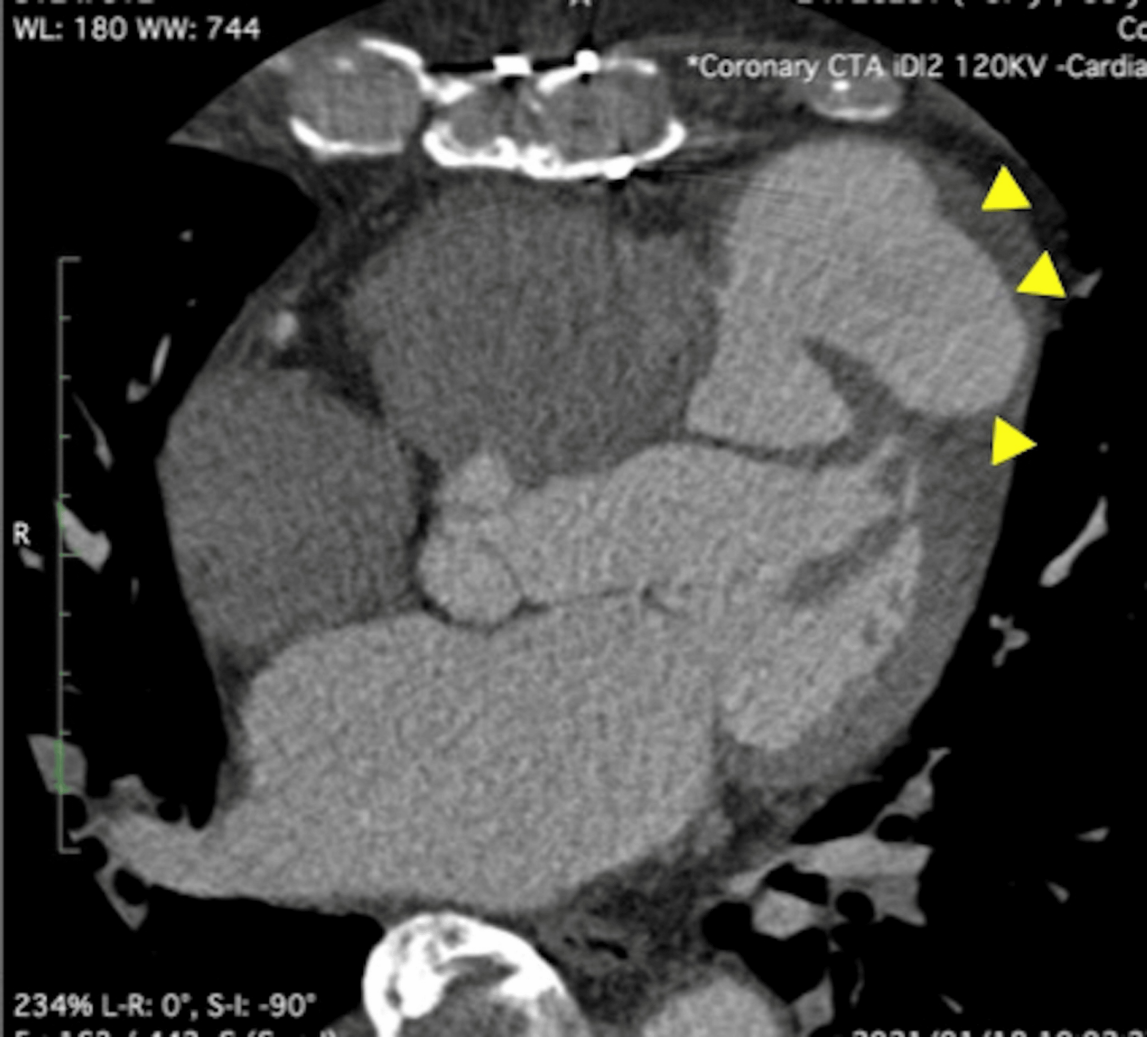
Cardiac computed tomography demonstrating pseudoaneurysm (yellow triangles) in the left ventricle
